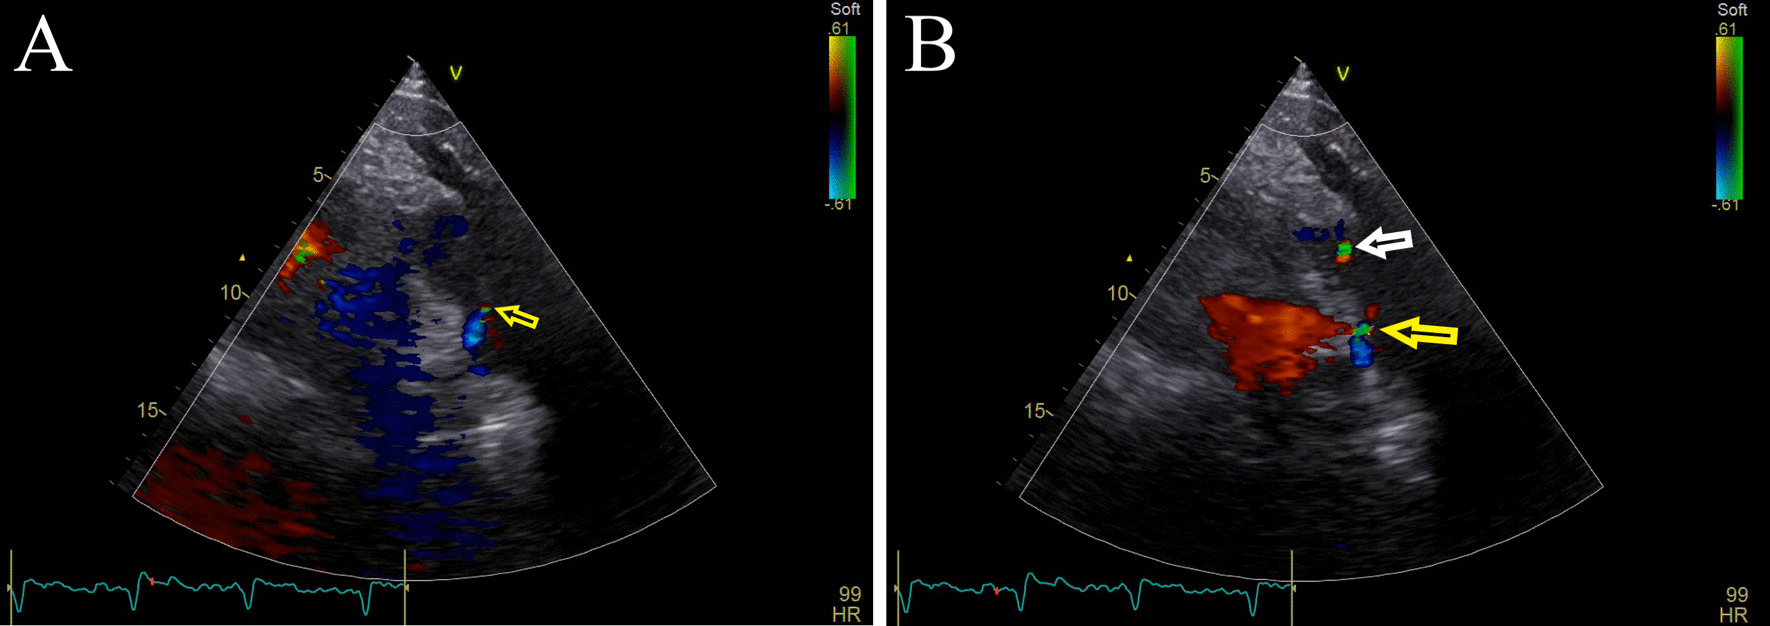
Echocardiography. A Parasternal right ventricular outflow view, abnormal flow during systole (yellow arrow). B Abnormal flow during diastole (yellow arrow) synchronous to pulmonary insufficiency jet (white arrow)
